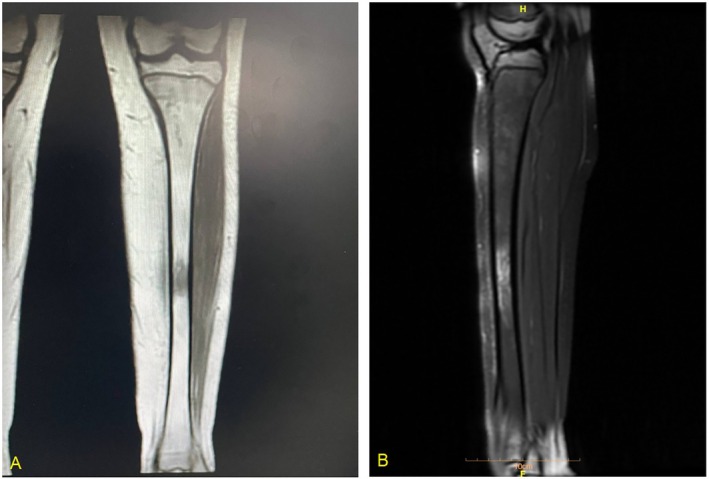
MRI of the left tibia demonstrating an active LCH‐associated osteolytic lesion. Coronal MRI of the left tibia showing (A) a well‐demarcated hypointense lesion on T1‐weighted imaging and (B) corresponding hyperintense signal on T2‐weighted imaging, consistent with an active osteolytic lesion in LCH.
